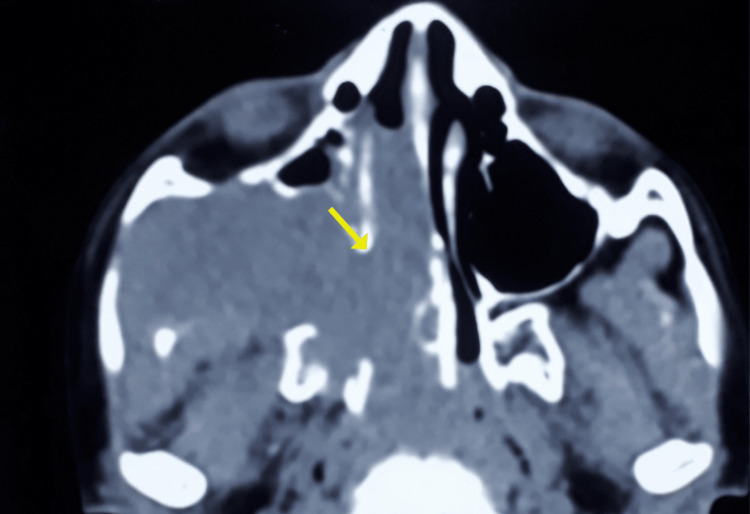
Contrast-enhanced computed tomography of paranasal sinuses showing bony resorption
